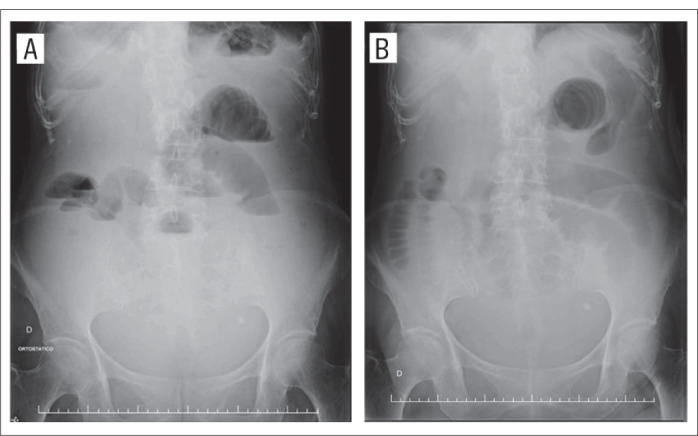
Abdominal X-ray. A and B: small bowel distention.
